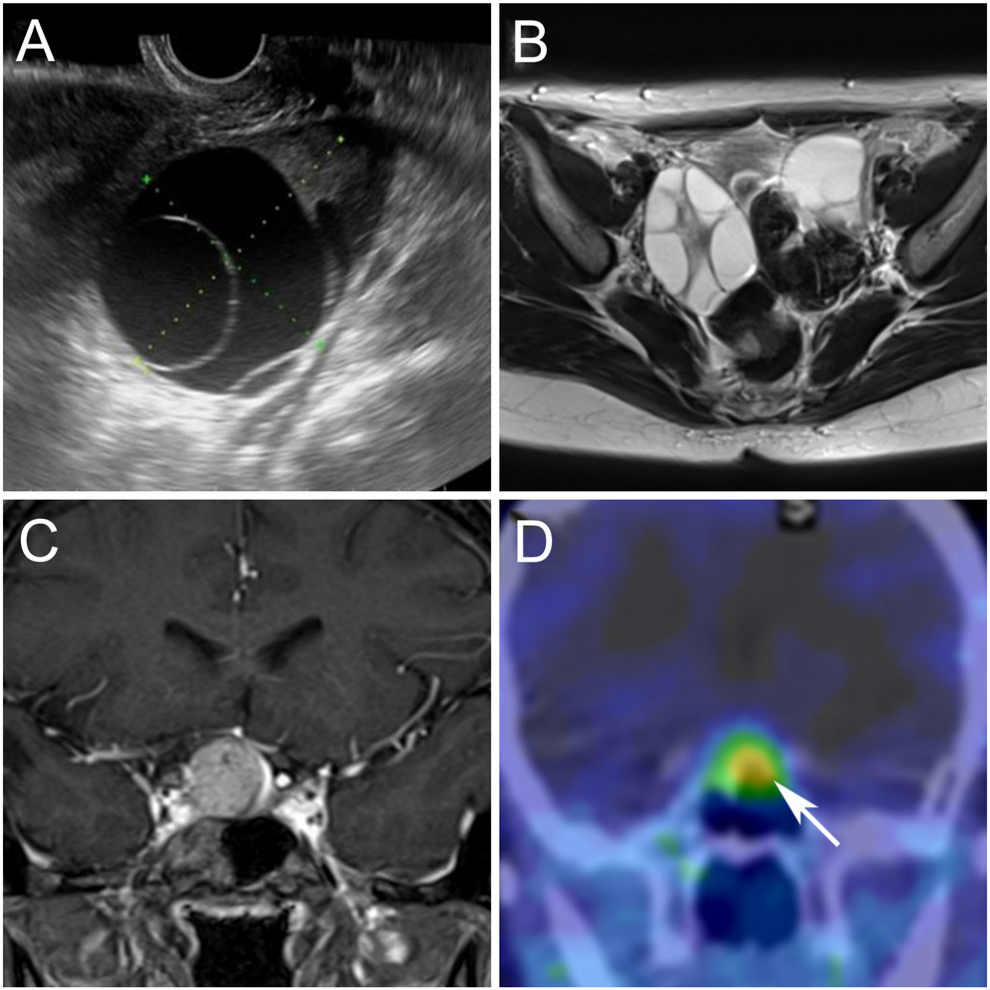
Preoperative imaging findings of ovary and pituitary lesions. (A) Transvaginal ultrasound showed a large septated cystic ovary. (B) Pelvic MRI (axial T2-weighted image) showed bilateral multi-cystic ovaries. (C) preoperative pituitary MRI (coronal postcontrast T1-weighted image) revealed a mass lesion with suprasellar extension. (D) Preoperative ^111^In-pentetreotide scintigraphy showed increased tracer uptake in the pituitary lesion (arrow).
